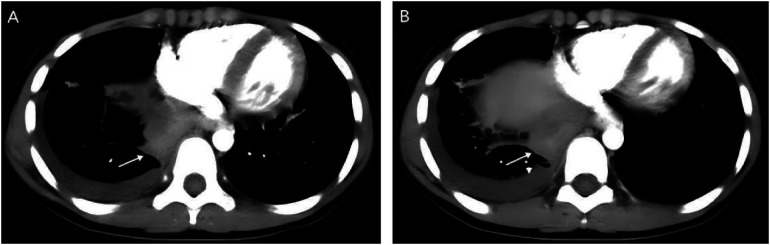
Contrast-enhanced chest CT. (A) A soft-tissue mass (arrow) with ill-defined margins, adherent to the adjacent lung parenchyma. (B) A soft-tissue mass (arrow) adherent to the diaphragm with associated pleural effusion.
